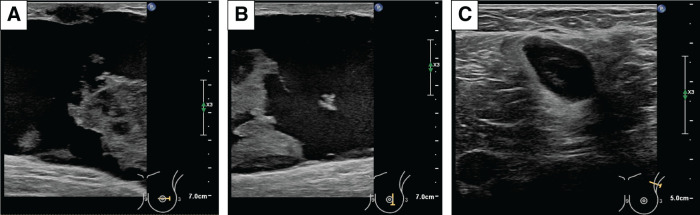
US at NAC midpoint (A: central portion of left breast tumor; B: caudal marginal portion of left breast tumor; C: Cystic changes of axillary lymph nodes). Tumor and lymph node size reduction is noted. NAC, neoadjuvant chemotherapy; US, ultrasonography
